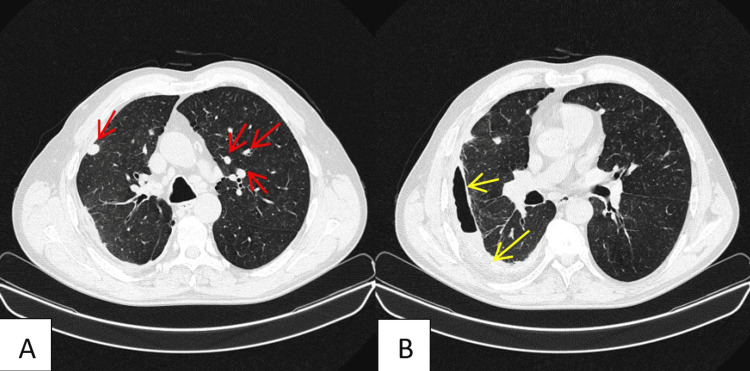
Chest CT scan A: Chest CT showed multiple solid nodules in both lungs (red arrows). These were distributed centrally and peripherally, but most of them were subpleural located. The largest nodules are located in the anterior segment of the left upper lobe, measuring 2.4 cm, and in the right lower lobe, measuring 2.2 cm. B: Right hydropneumothorax (yellow arrows) and mild parenchymal atelectasis are observed in the right lung. They are subpleural, adjacent to the effusion. The parenchyma presents a mild mosaic density pattern, suggesting ventilation-perfusion alterations. No new changes are identified in the lung parenchyma.
